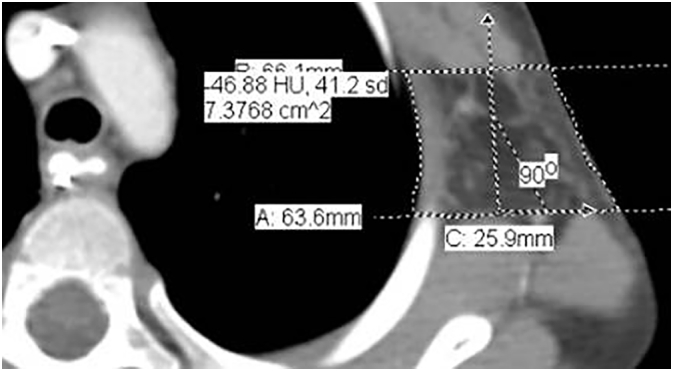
Cropped CT scan showing the left axilla with an equivalent width measurement. Two parallel lines define the margins of the pectoralis major and minor and the latissimus dorsi and subscapularis muscles. The lung surface and skin surface are defined with dotted lines connecting the two parallel lines. The equivalent width is 7.38 cm^2^/2.59 cm = 2.84 cm for the axillary depth. The line labeled 90° represents the normal between the two parallel lines along which the distance between the parallel lines is measured.
